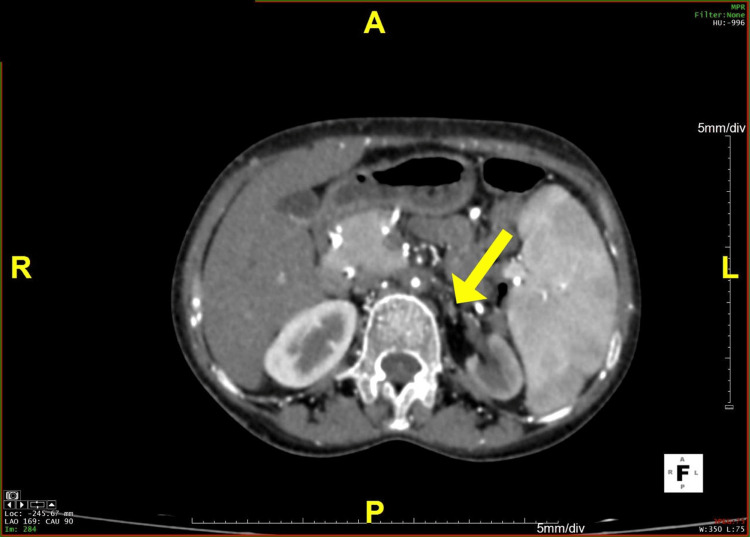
An axial section of the CT angiogram revealing severe stenosis with non-opacification of the left renal artery CT: computed tomography
